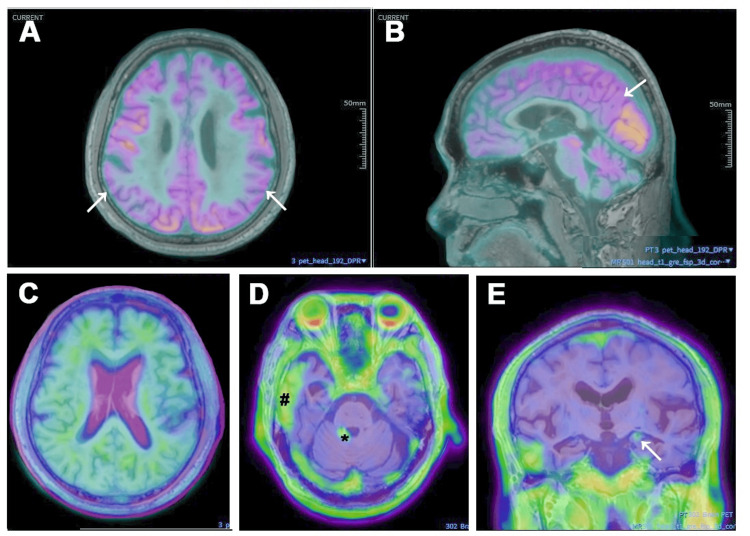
AD-related multimodal PET imaging (A-B) FDG PET demonstrates hypometabolism in bilateral precuneus and temporoparietal regions (arrows). (C) Amyloid PET (AV45) reveals widespread cortical Aβ deposition, consistent with AD pathology. (D-E) Tau PET highlights tau accumulation in the hippocampi (arrow), lateral temporal lobes (#), and cerebellum (*), consistent with AD pathology. AD: Alzheimer’s disease; FDG: Fluorodeoxyglucose; AV45: Florbetapir; Aβ: Amyloid-beta
